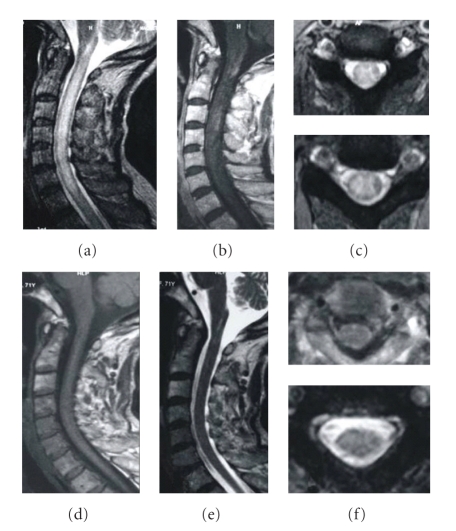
A 70-year-old female was admitted due to progressive paraparesis, paresthesias, and urinary retention for 2 months. After 2-year of ATT, her situation improved. The MRI changes are as follows: (a) sagittal T2-weighted image reveals a long, high intensity signal from C1 to T1 in the spinal cord before treatment regimen, (b) T1W contrast image showing patchy enhancement and thickening of the cord, (c) the diffuse lesions on axial T2-weighted images, (d) a year later the swelling of the cervical spinal cord disappeared on sagittal T1-weighted images, (e) the hyperintensity resolved following 1 year of ATT, and (f) no lesion is visible on axial T2-weighted images.
